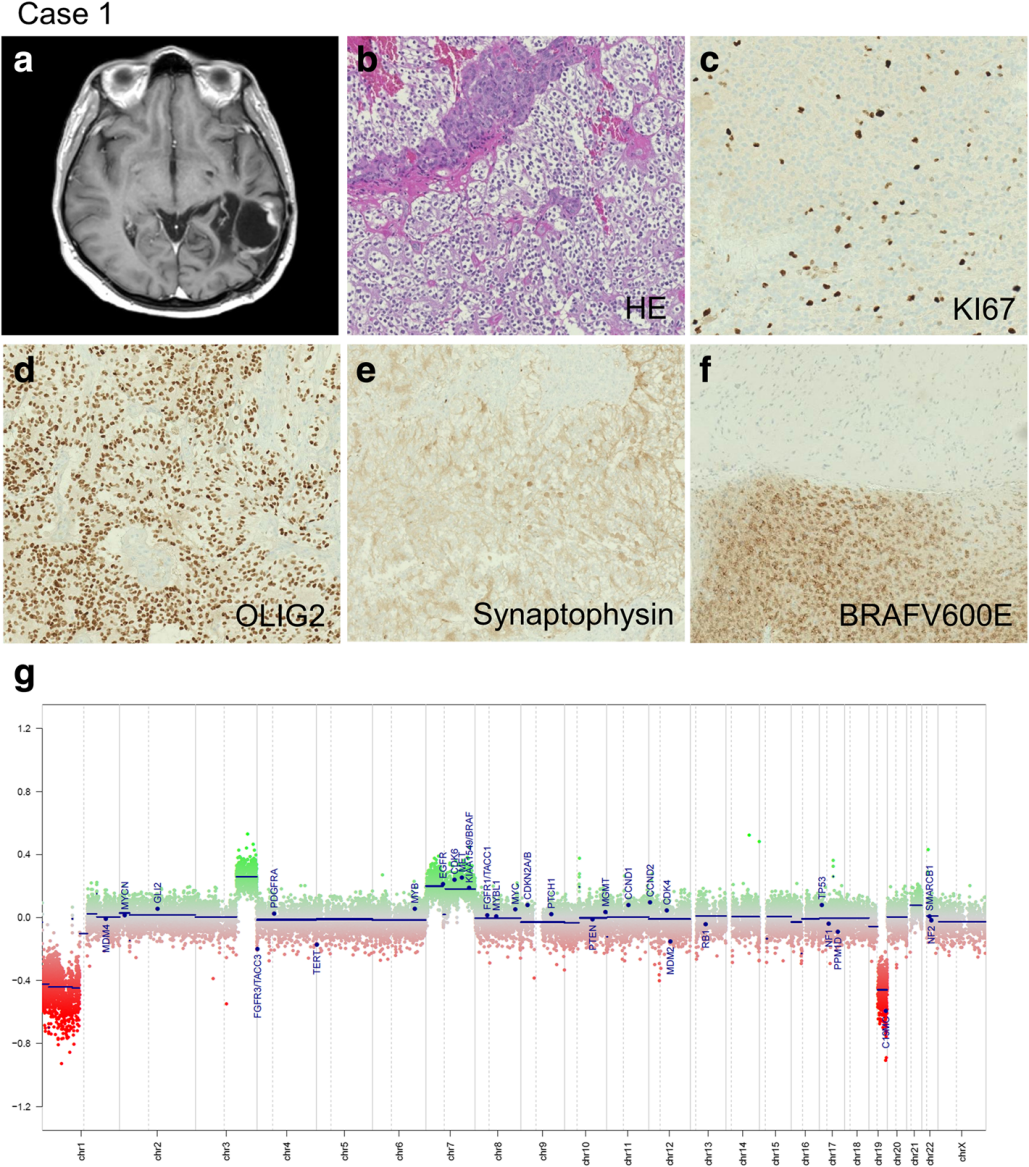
a MRI disclosed a nodule enhanced after gadolinium injection, located within the intraoperative cavity. b High cellularity neoplasm composed of relatively monomorphic oligodendrocyte-like cells associated with endocrinoïd vasculature. c KI67 labeling index was around 10%. d, e, f Tumor cells strongly expressed OLIG2 and synaptophysin and BRAFV600E. g Copy-number profile derived from DNA-methylation arrays revealed 1p/19q codeletion with additional gains of chromosomes 3 and 7
